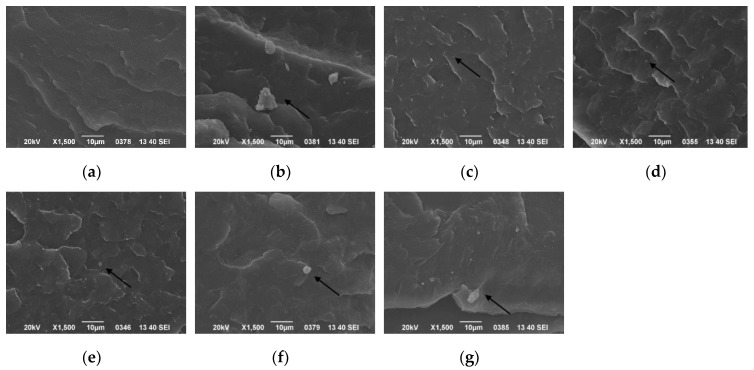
Effect of different ultrasound distances on dispersion of the nucleating agent in the PP matrix: (a) pure PP; (b) without ultrasound; (c) 1 cm; (d) 2 cm; (e) 3 cm; (f) 5 cm; (g) 7 cm.
